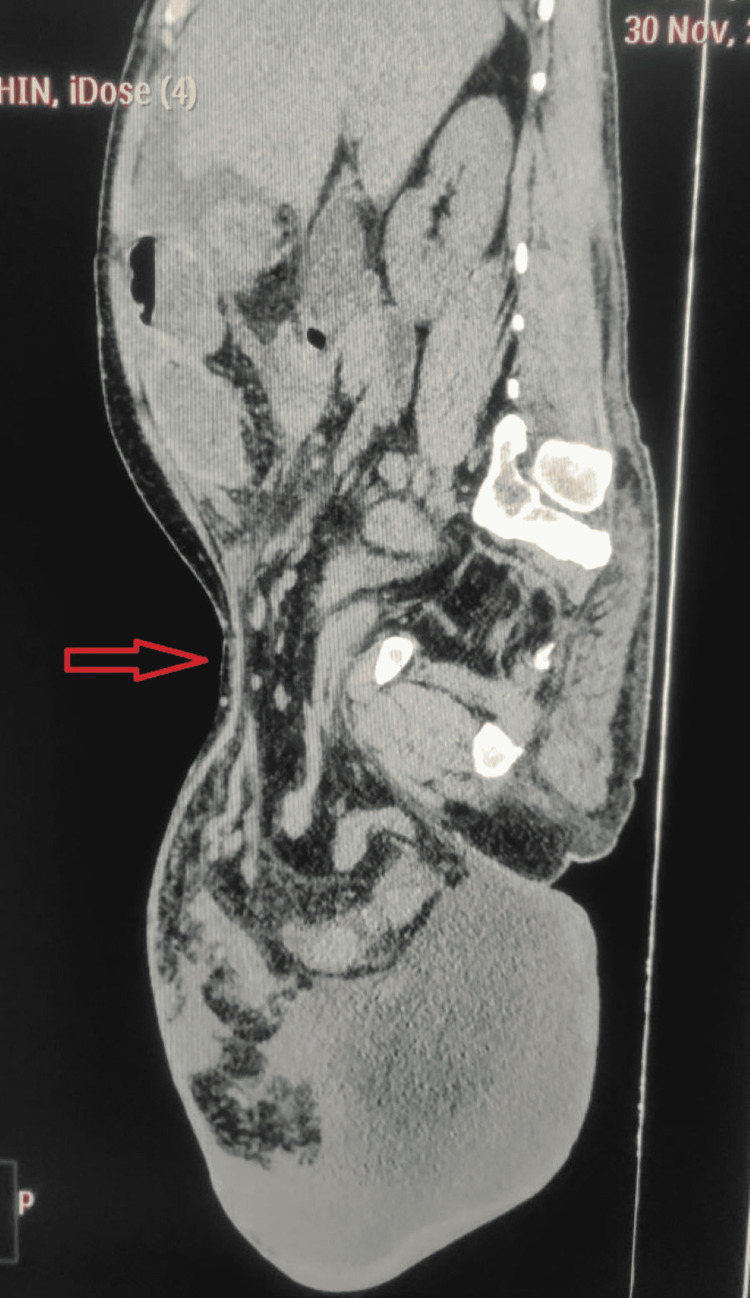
Noncontrast CT sagittal view depicting herniation of the abdominal contents into the scrotum. CT: computed tomography
